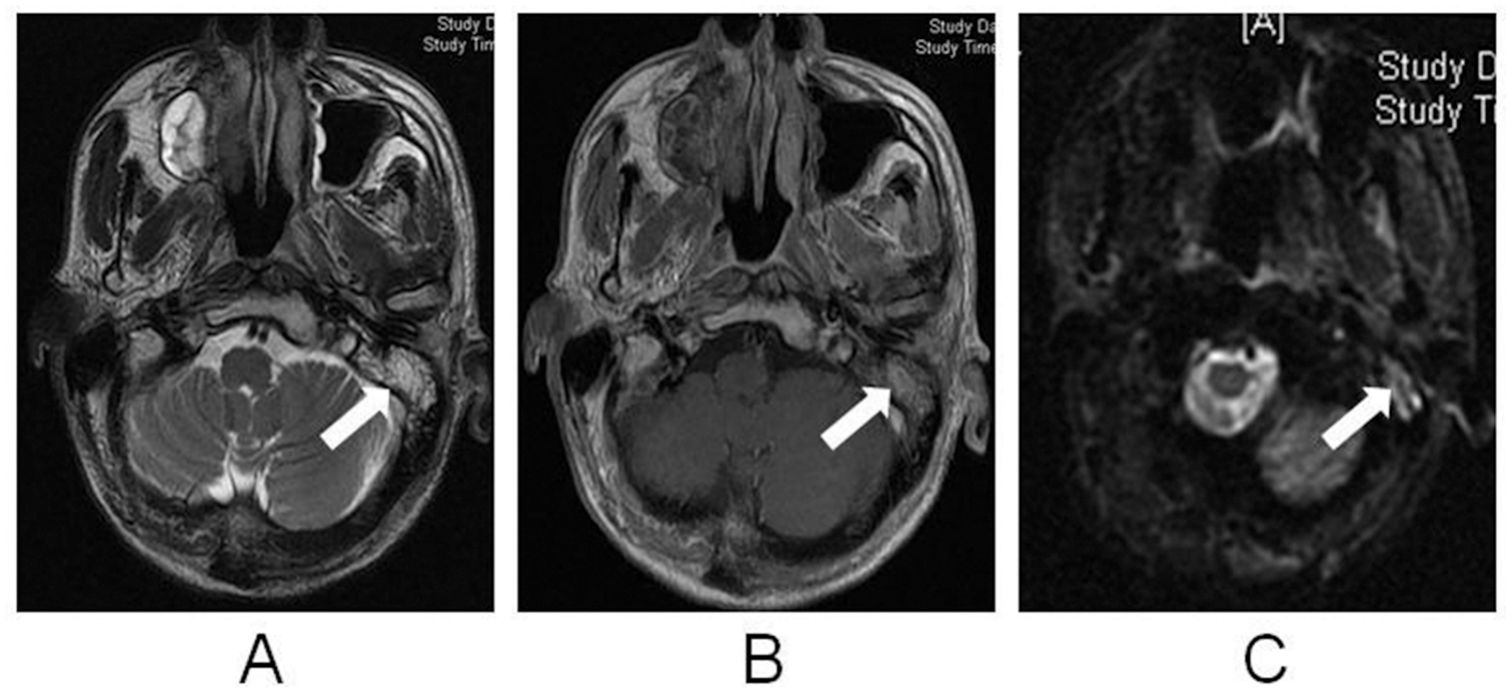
MRI images of a 45-year-old male patient with nasopharyngeal cancer and mastoiditis in the left ear. (a) Axial T2W image; (b) T1W contrast-enhanced image with fat saturation; (c) DWI (*b* = 1000). Fluid accumulation with peripheral contrast enhancement and restricted diffusion is observed in the left mastoid (white arrow); the right mastoid appears normal.
